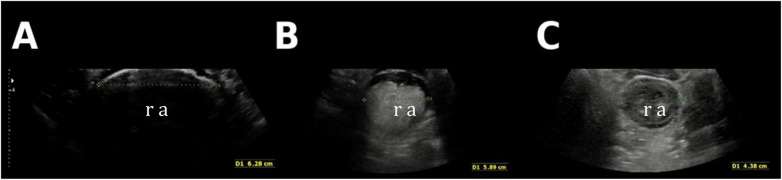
A 12-year-old girl with cerebral palsy and chronic constipation. Serial transabdominal ultrasound demonstrates persistent rectal dilatation despite treatment: (A) initial examination with a transverse rectal diameter of 6.28 cm, (B) follow-up examination after treatment showing a transverse diameter of 5.89 cm, and (C) subsequent follow-up with a transverse diameter of 4.38 cm. r a: rectal amulla.
